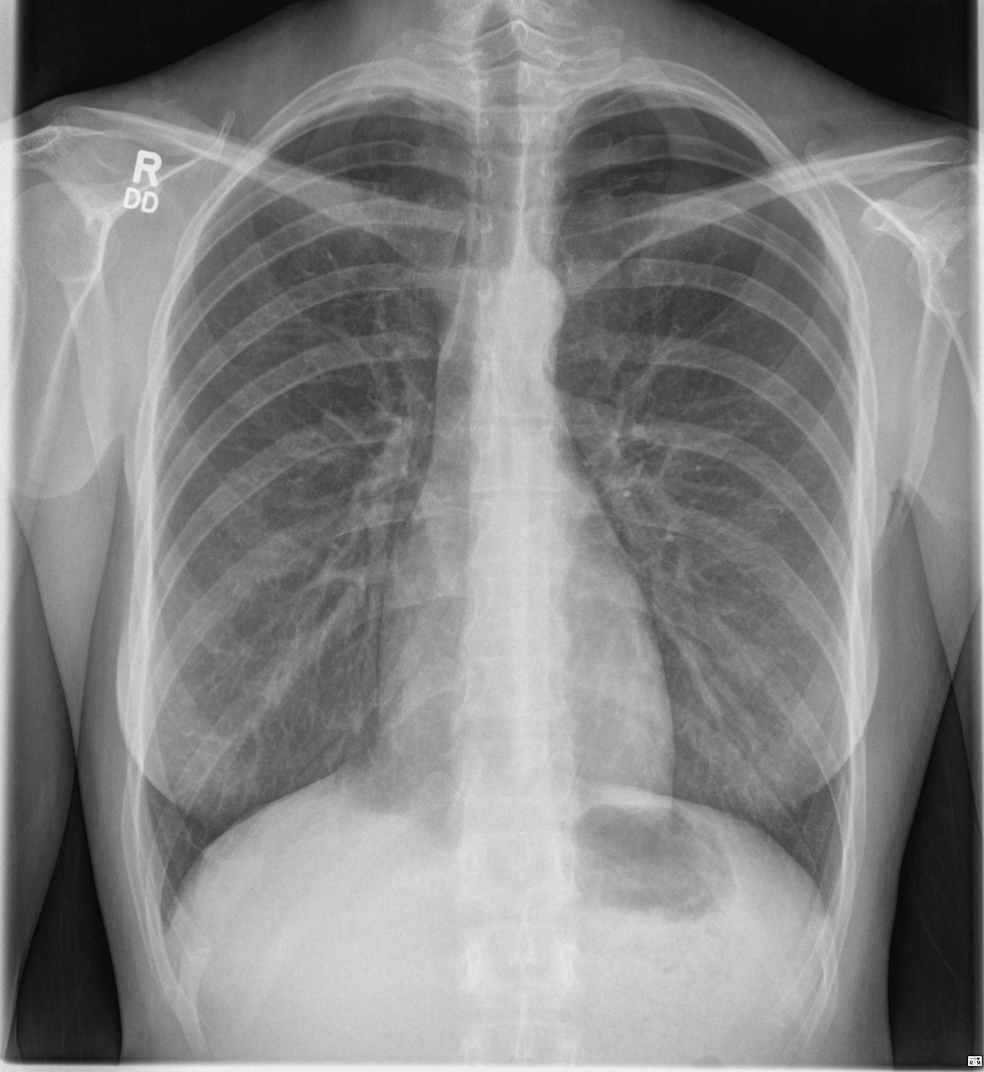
Patient’s chest radiograph, interpreted as showing no abnormality.
